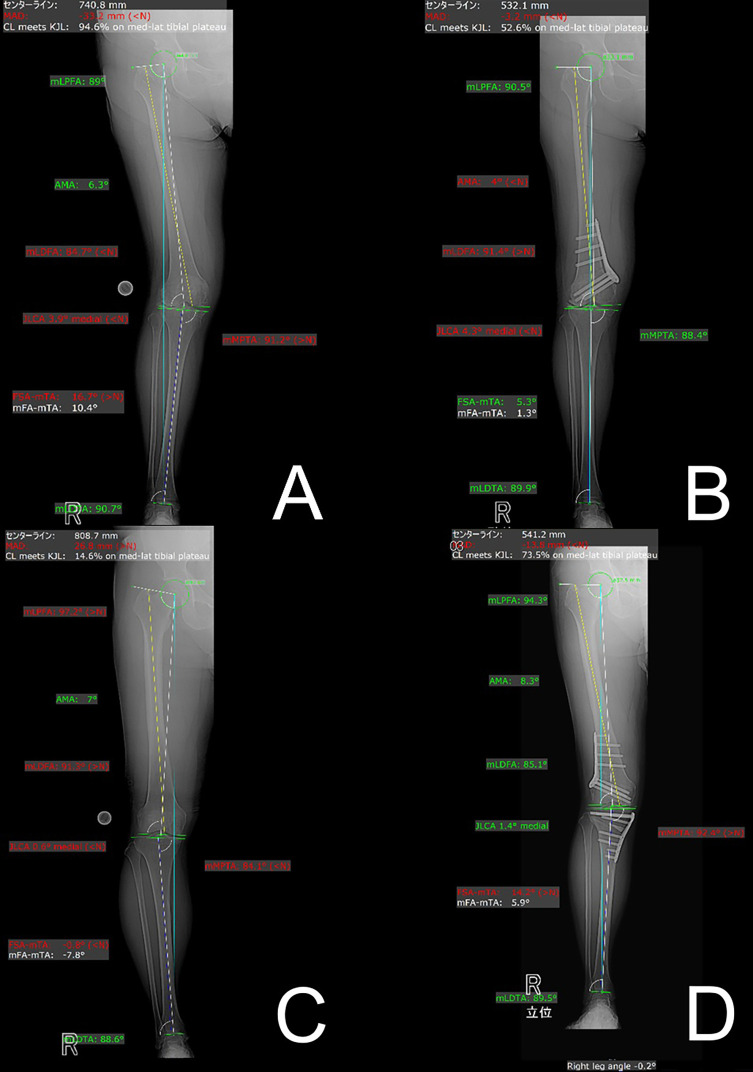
A. Preoperative full-length standing radiograph of the lower limb after MCWDFO for genu valgum. The mLDFA was 84.7°, demonstrating valgus alignment originating from the distal femur. B. Postoperative full-length standing radiograph of the lower limb after MCWDFO. The mLDFA was corrected to 91.4°, and the overall valgus alignment was corrected. C. Preoperative full-length standing radiograph of the lower limb after LCWDFO for genu varum. The mLDFA was 91.3° and mMPTA was 84.1°, demonstrating varus alignment originating from the distal femur and proximal tibia. D. Postoperative full-length standing radiograph of the entire lower limb after LCWDFO. The mLDFA was corrected to 85.1° and mMPTA was corrected to 92.4°, and the overall varus alignment was corrected.
